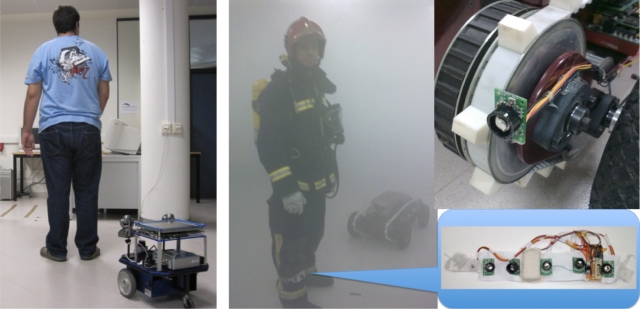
Robot following a human using computer vision (left). Robot following a firefighter using ultrasound and radio sensors. An ultrasound ring transmitter is attached to the firefighter’s leg. The robot can follow the firefighter using the received signals (right).
